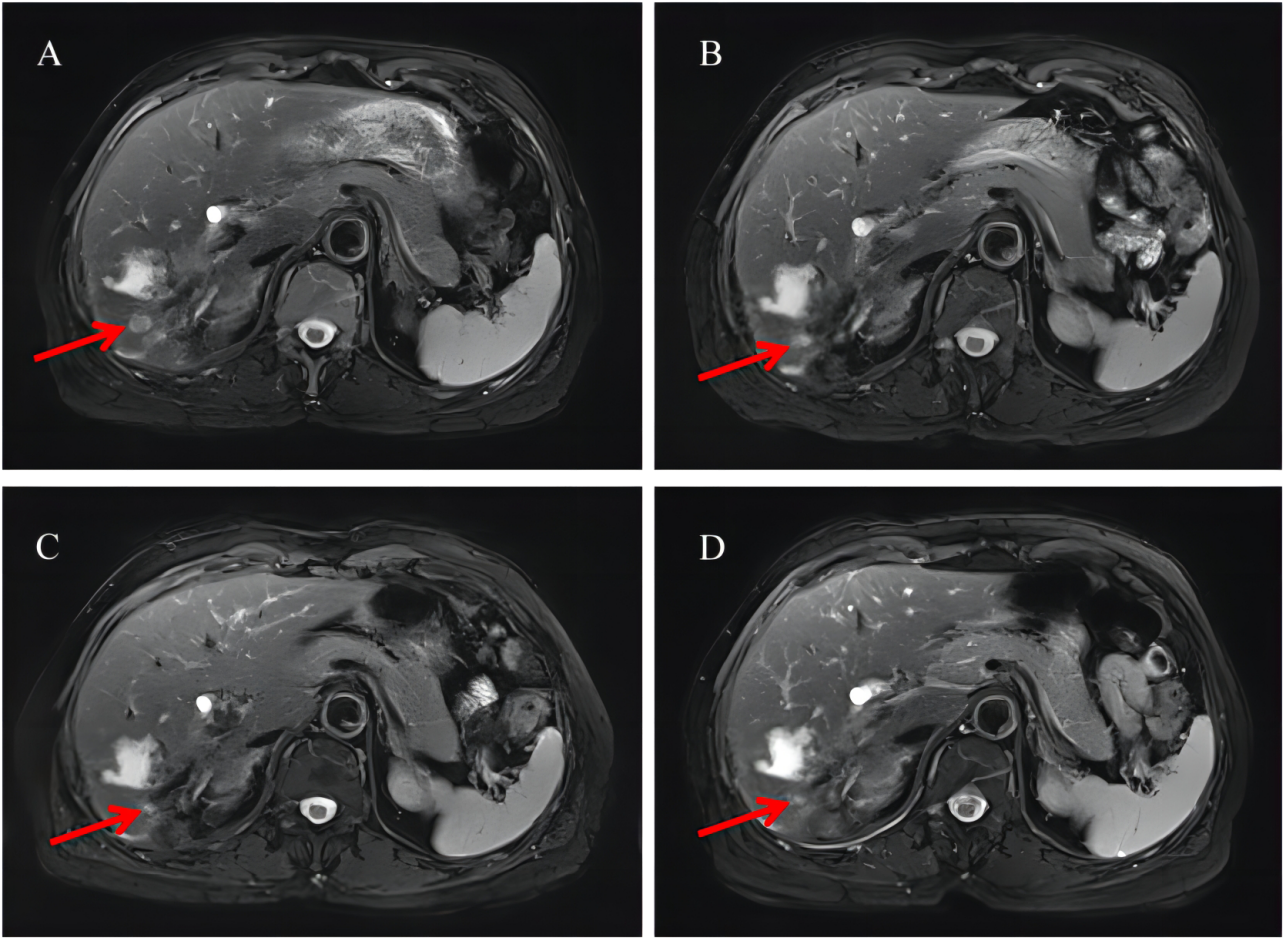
Radiological examination of the patient. (A) Liver MRI after one cycle of chemotherapy. (B) Liver MRI after combination therapy for 2 months. (C) Liver MRI after combination therapy for 4 months. (D) Liver MRI after combination therapy for 8 months. MRI, magnetic resonance imaging. The red arrow in the figure points to the metastatic tumor in the right posterior lobe of the liver.
